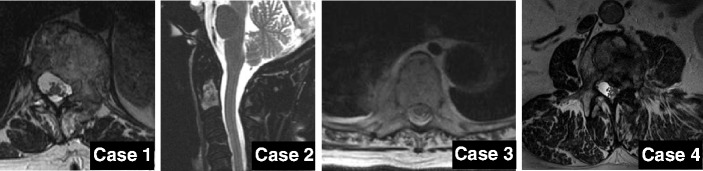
Representative slices from T2 weighted MR images of all patient cases. Case 1,3,4 are presented in axial view and case 2 in sagittal view
